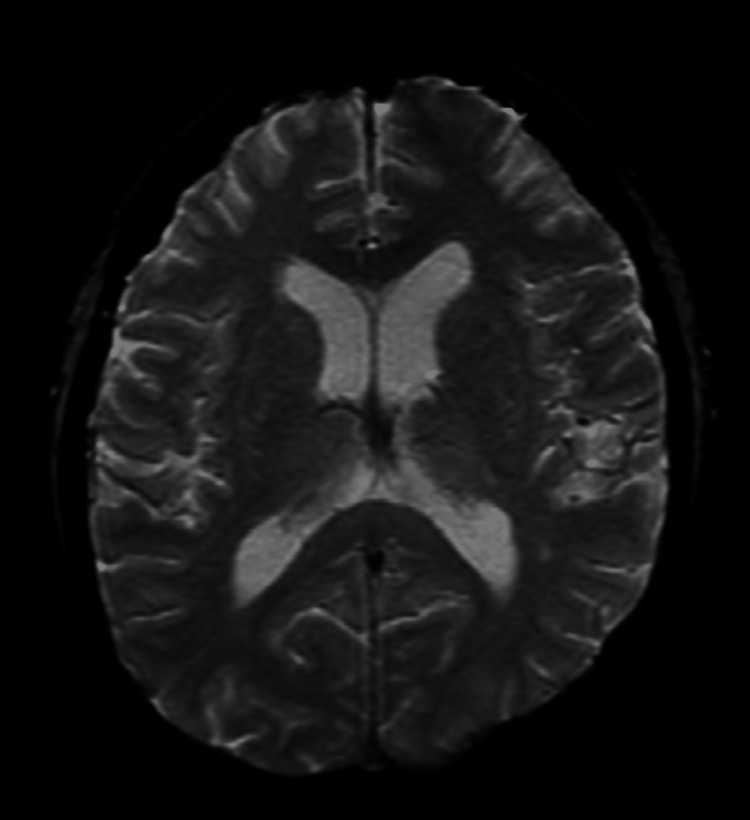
No evidence of hemorrhage Finding: Axial MRI of the brain GRE sequence showing no evidence of hemorrhage. Technique: 1.5 T MRI, GRE. MRI: magnetic resonance imaging; GRE: gradient-recalled echo
